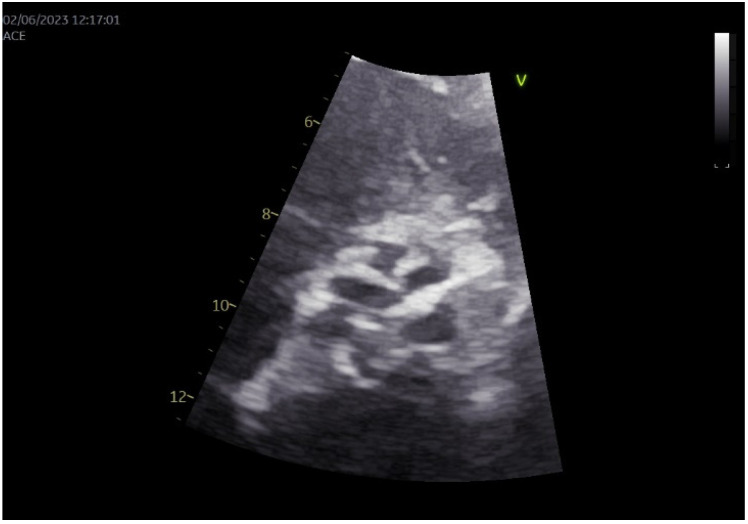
A focused transthoracic echocardiographic examination using GE^®^ Vivid E95 (GE HealthCare, Chicago, IL, USA) in the short-axis view demonstrated a heavily calcified aortic valve.
